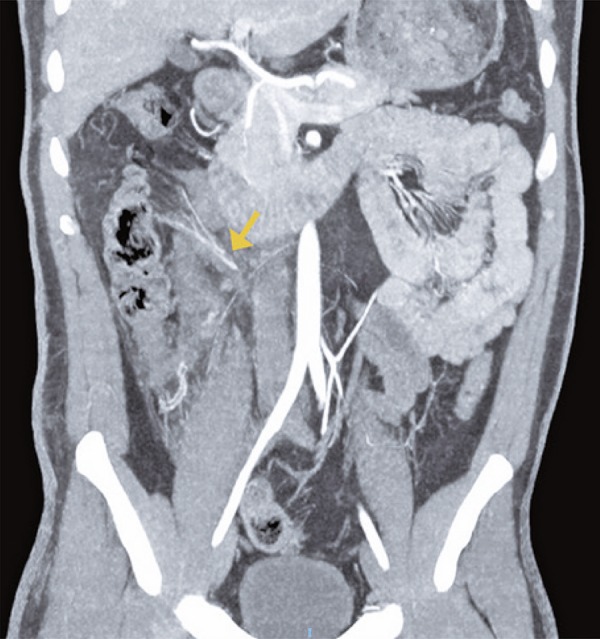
Coronal reconstruction of computed tomography in angiographic phase in maximum intensity projection, showing parietal irregularity and hypoenhancement in the middle and distal segments of a right colic vessel branch (arrow)
